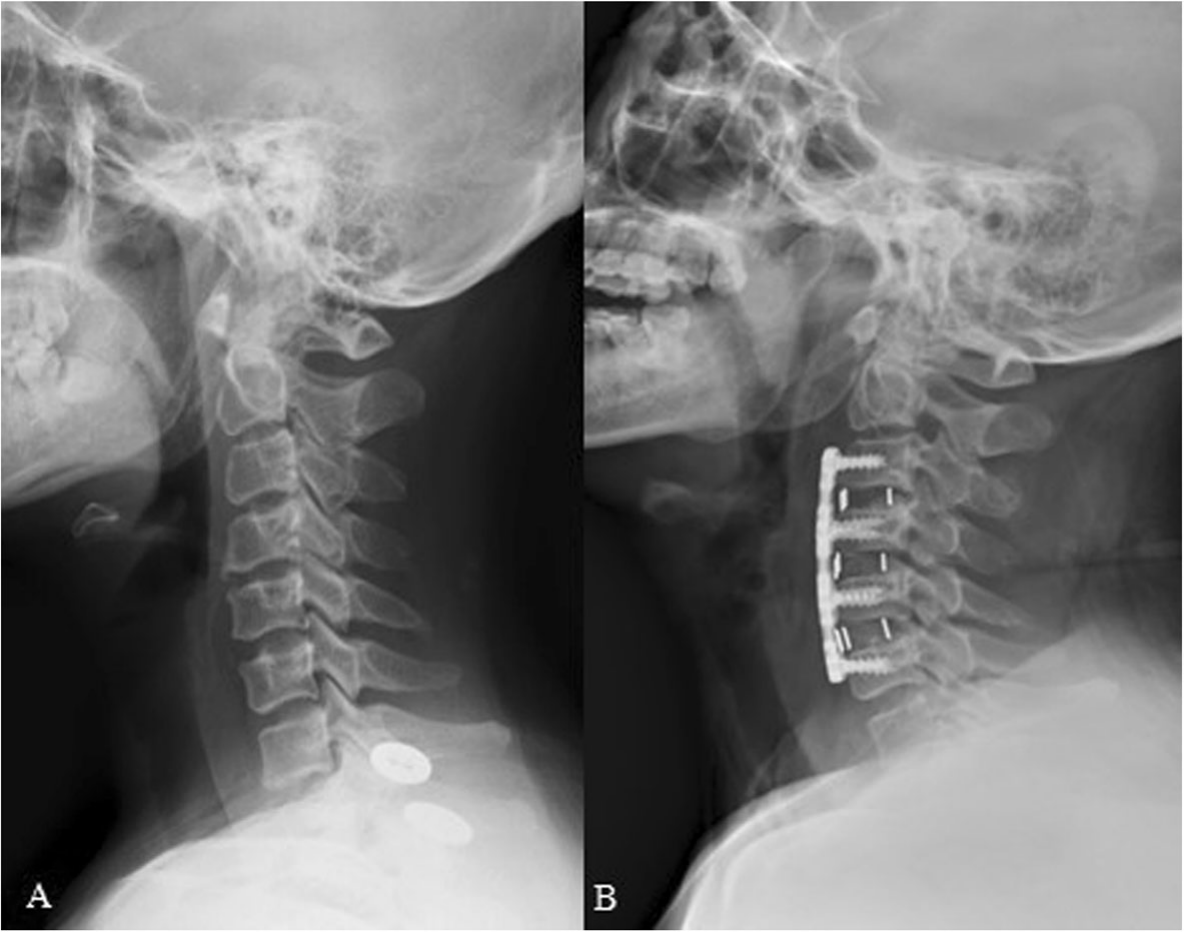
Multi-level ACDF was performed to release the compression. Lateral X-ray of cervical spine was taken in a 56-year-old male patient with MCSM at preoperative (a) and 2-year follow-up visit (b) and sagittal parameters were corrected appropriately
